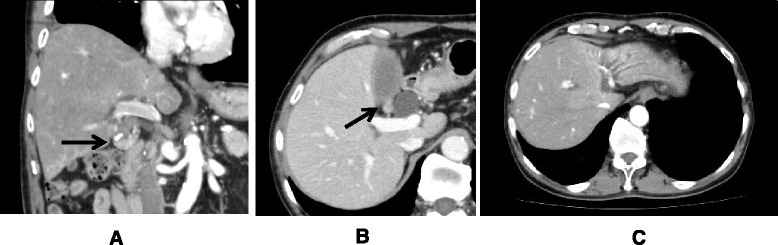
Coronal section of computed tomography indicated contrast-enhanced wall thickness of the neck of the gall bladder (a) and axial section showed swelling of lymph node measuring 1.2 cm in diameter (b). The dilated intrahepatic bile duct was seen in left liver (c); this finding was consistently observed in the patient’s clinical course and indicated no malignant findings
